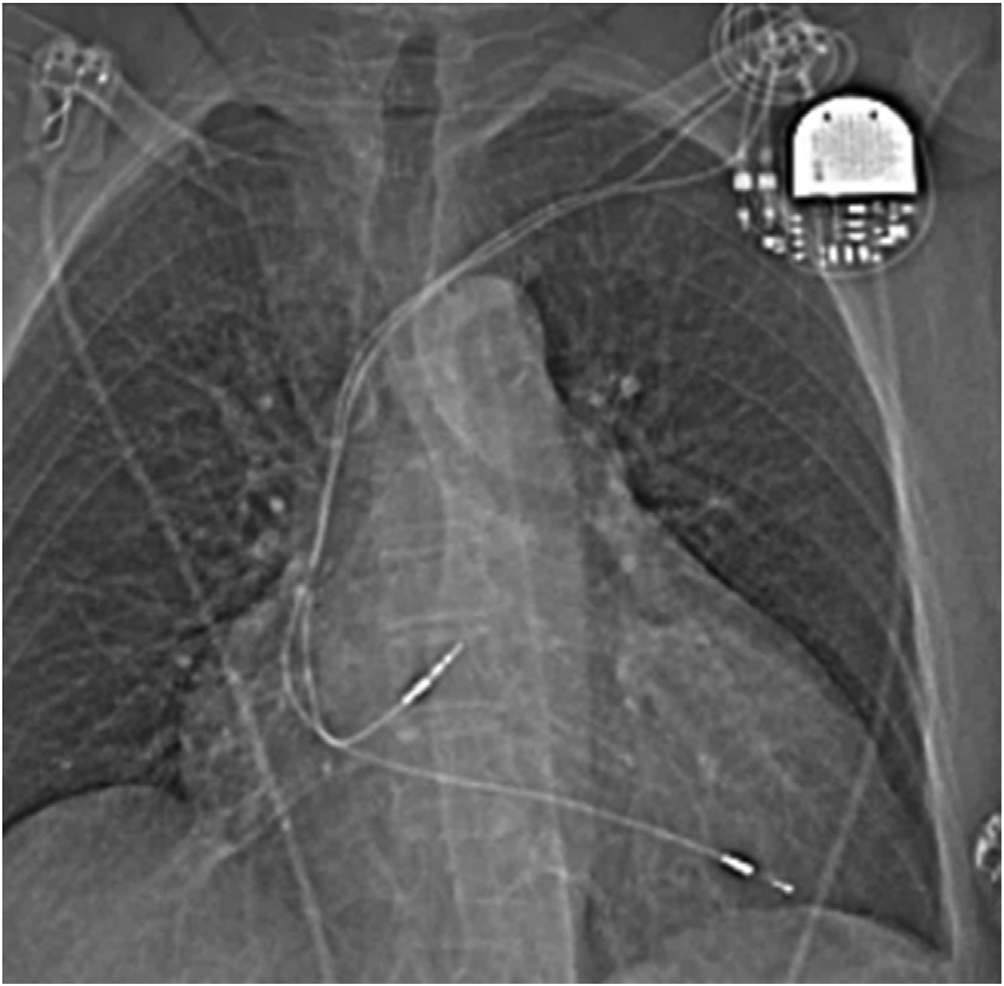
Patient X-ray showing the presence of the leads and pacemaker.
